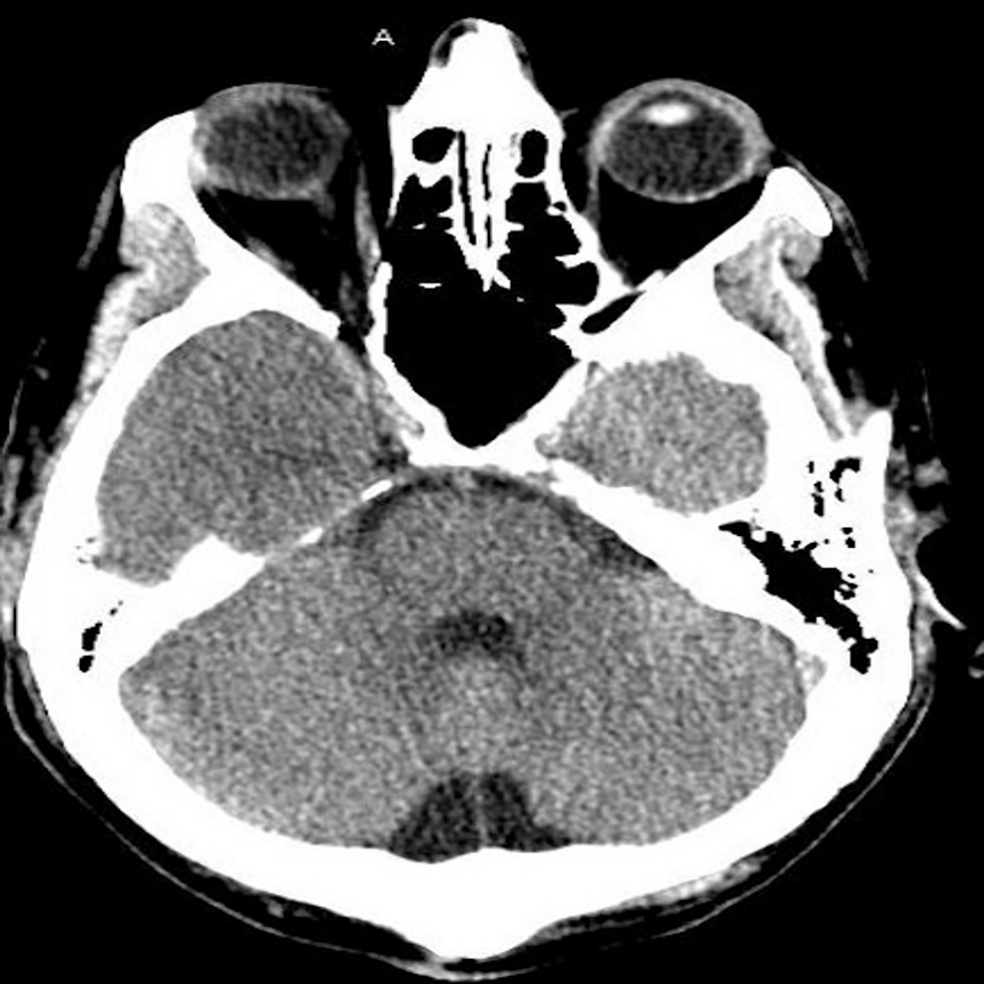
CT scan of the head without contrast. Unremarkable CT scan of the head showing no intracranial pathology.
